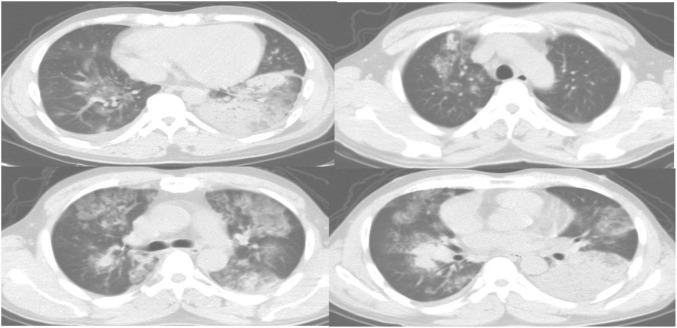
CT images of a 51-year-old male patient.
